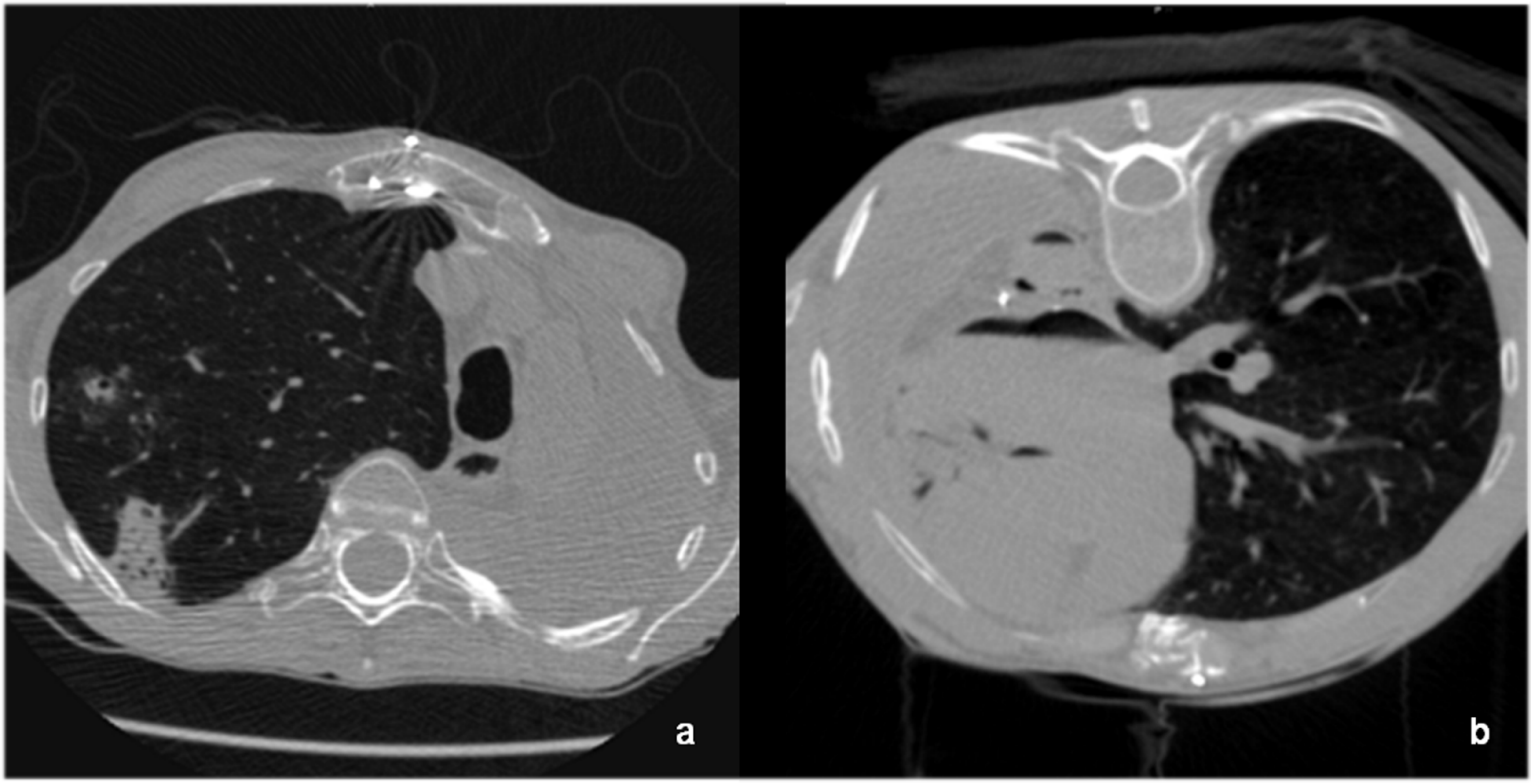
a and b: Transversal CT-slice, showing SAE in the coronary arteries, the aorta, the left atrium and ventricle (a). The lesion was located in the left upper lobe (b), at the time of biopsy approximately 8 cm above the level of the left atrium. 49 y/o patient after lung transplantation, suffering from pulmonary aspergillosis. She died due to coronary ischemia.
